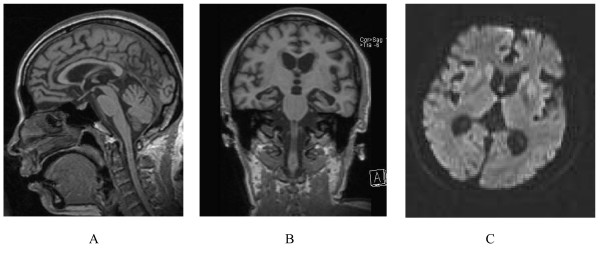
(A and B) Magnetic resonance imaging, showing clear bilateral atrophy of cortex, brainstem and cerebellum. (C) Diffusion weighted imaging, displaying high signal in the caudate nucleus and putamen.
